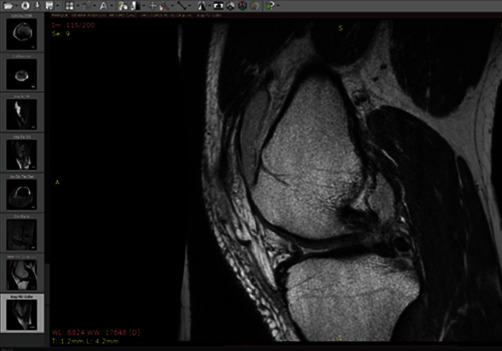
Sequence of cuts of a knee by means of an MRI.
